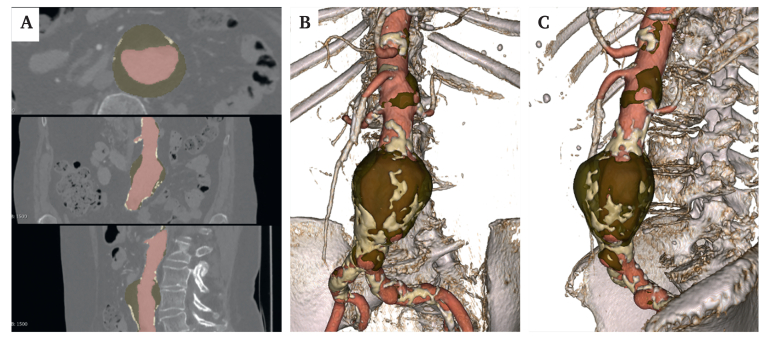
Representative images of the segmentation of the arterial lumen in red, intraluminal thrombus in brown, and parietal calcifications in light yellow. A Computed tomography angiography multiplanar sections from a patient with an infrarenal abdominal aortic aneurysm annotated with the fully automated segmentation. B, C Frontal and left sagittal views of the 3D aorto-iliac automated segmentation reconstructions.
